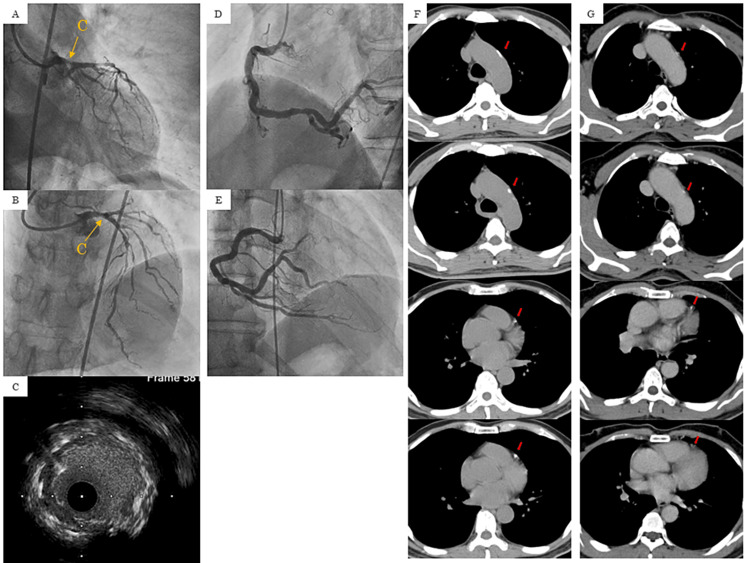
(A, B, C) When a drug-eluting stent was deployed into the LMT to LAD, TIMI grade 3 flow was obtained. Diffuse advanced stenosis was observed in LAD. Thrombus formation in a part of the stent was observed in angiography (yellow arrow) and IVUS. (D, E) Right coronary angiography did not reveal significant stenosis. (F, G) On day three, CT showed exacerbated findings of calcification in the LAD and aorta compared to before capecitabine was administered (red arrow). (C) IVUS findings, (F) on day 3, (G) before capecitabine was administered. Taken in the prone position. LMT: left main trunk, LAD: left anterior descending artery, TIMI: thrombolysis in myocardial infarction, IVUS: intravascular ultrasound, CT: computed tomography
